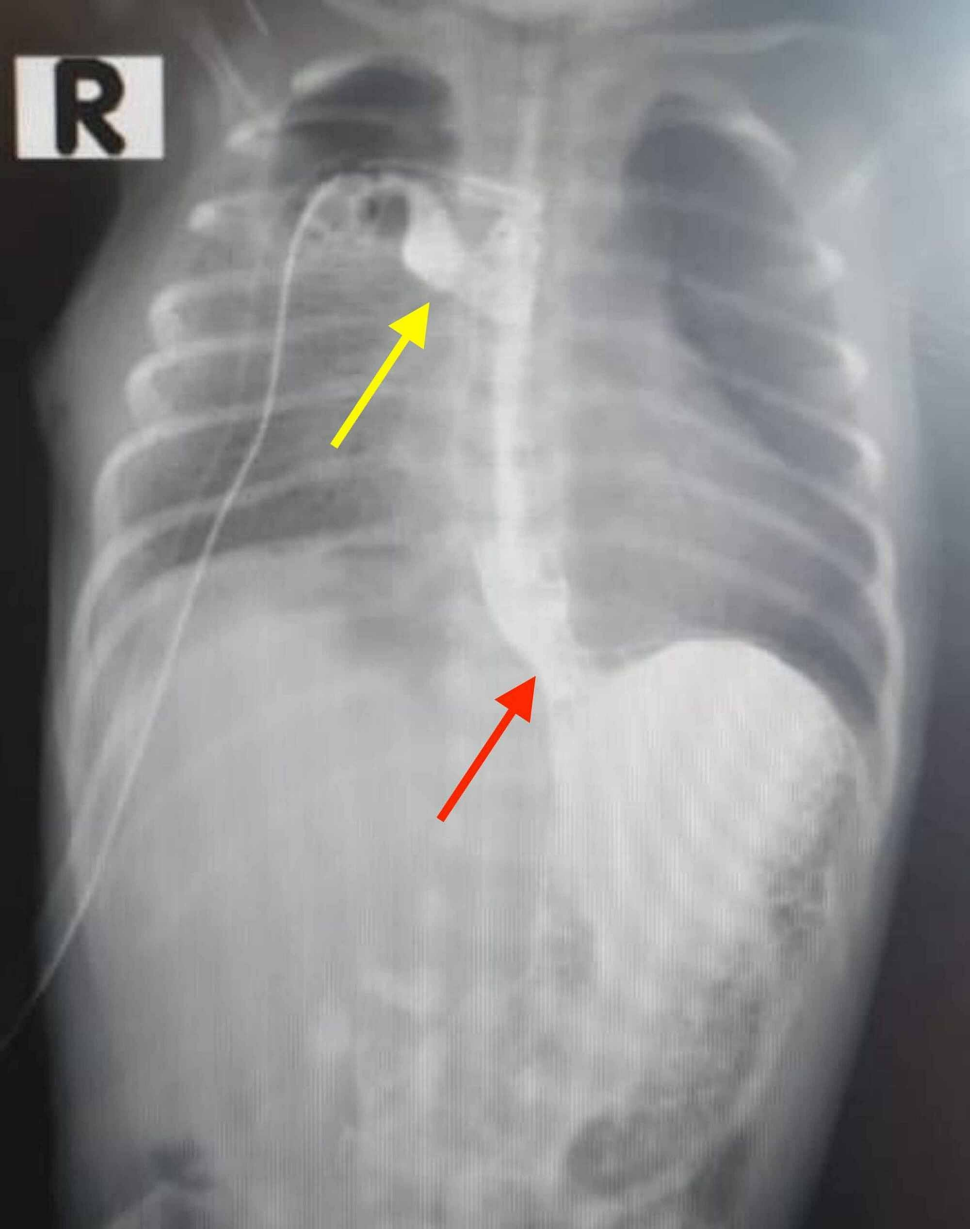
A postoperative radiograph showing minor leakage of the contrast from the anastomosis after the surgical repair of EA with TEF (yellow arrow). Gastroesophageal reflux is also noted (red arrow). EA with TEF: esophageal atresia with tracheoesophageal fistula.
